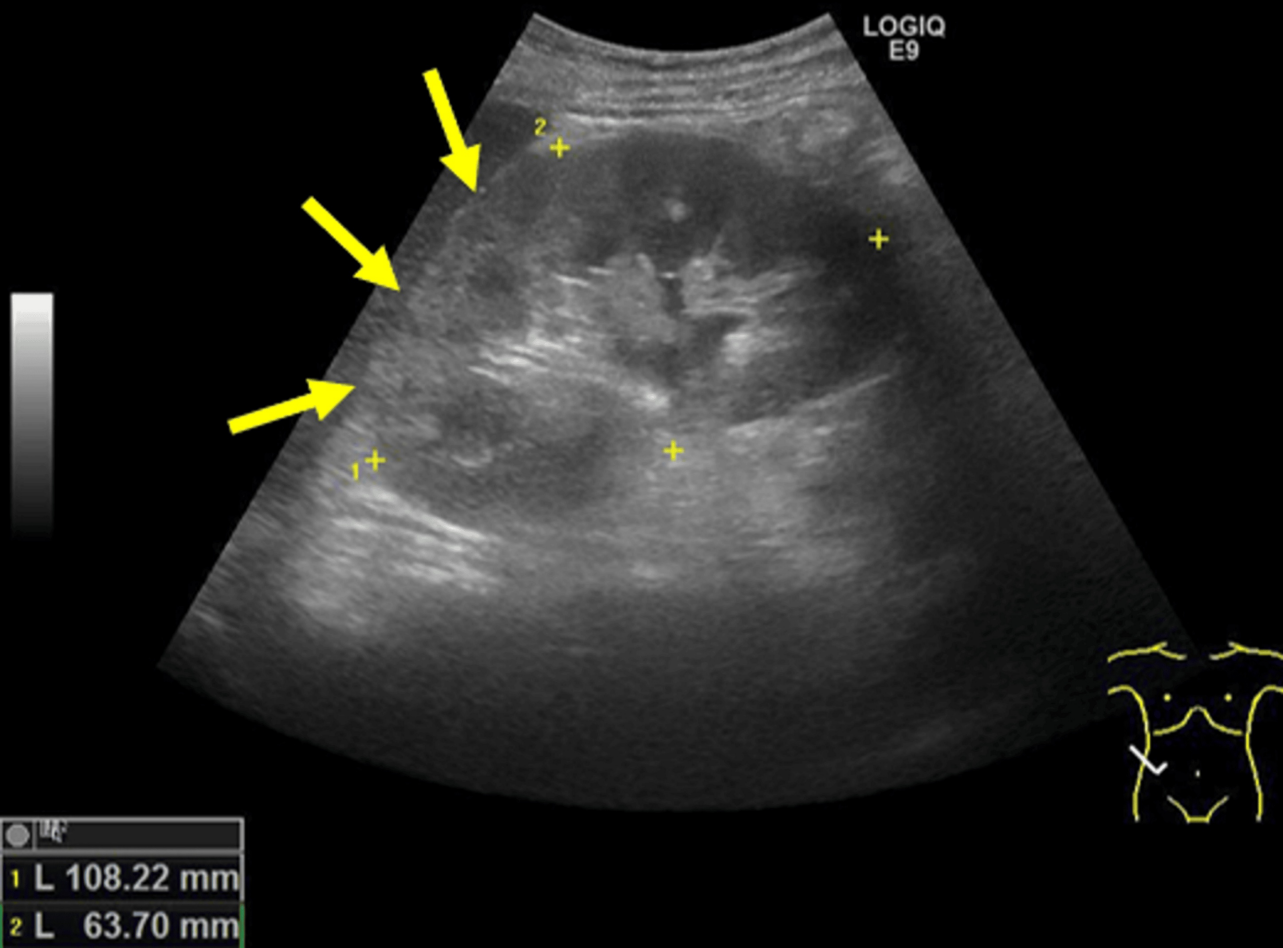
Color-Doppler-enhanced ultrasonography of the abdomen demonstrating a hyperechoic focal lesion in the right kidney
